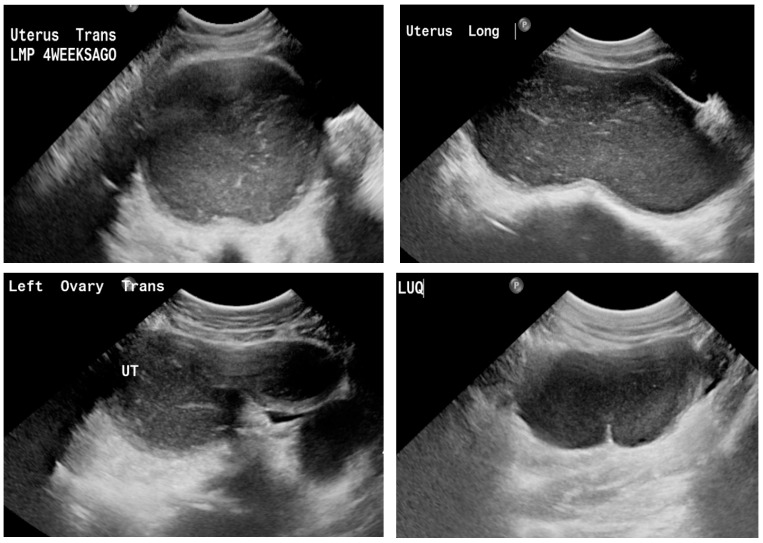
Transabdominal pelvic ultrasound (axial and longitudinal views) demonstrating a markedly distended fluid-filled vagina consistent with hematocolpos in a 15-year-old girl (Case 2).
